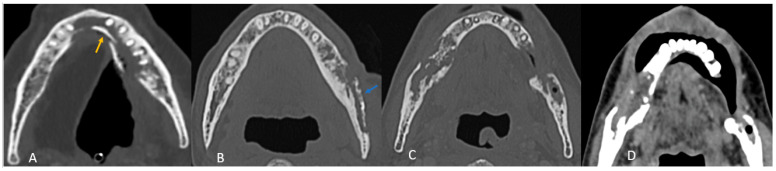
Axial CT images showing patterns of bony involvement in ORN. (A) Maximum bone alterations along the lingual cortex (with fragmentation—orange arrow) in a treated case of tongue carcinoma. (B) Maximum changes seen along the buccal cortex in a treated case of buccal carcinoma. (sequestrum—blue arrow). (C) Treated case of carcinoma of the base of tongue with ORN involving the posterior aspect of the body and the angle of the mandible. (D) Treated case of oropharyngeal carcinoma with ORN at both the angles of the mandible and iso-enhancing soft tissue at the site of bony involvement.
